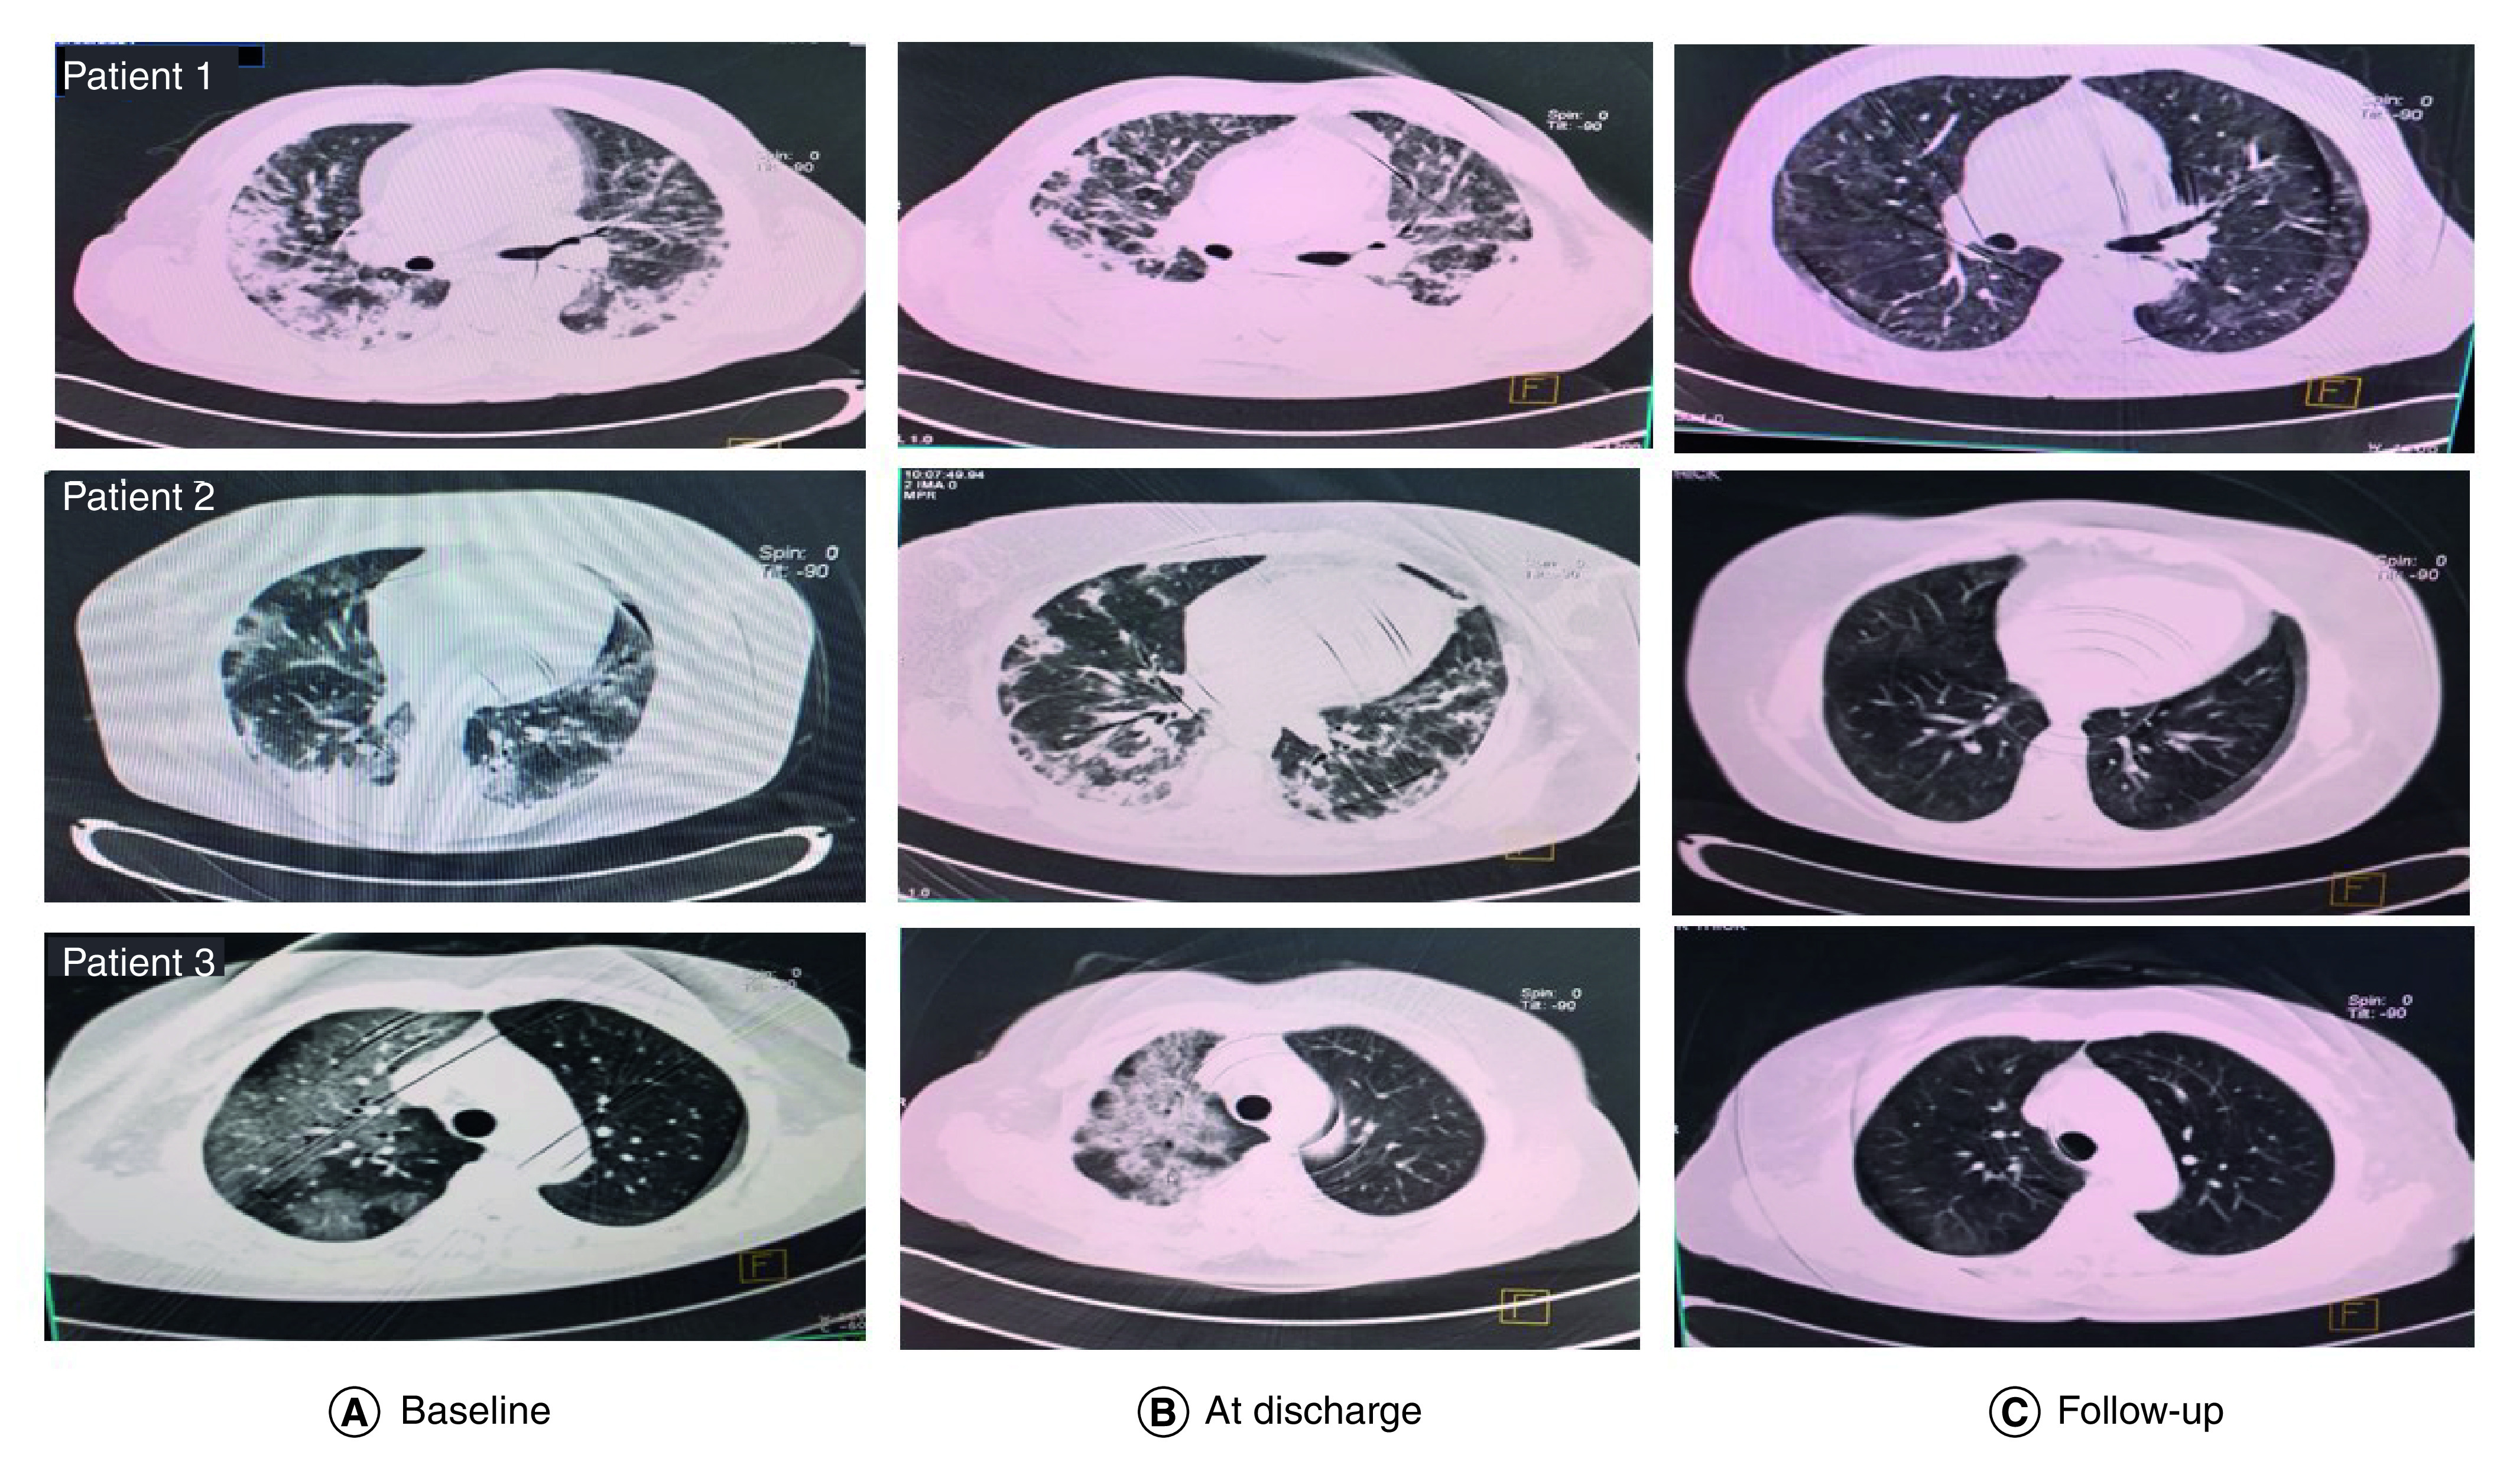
Axial chest CT scans of three patients treated with nimotuzumab. Sequential images on admission (column A), at discharge (column B) and follow-up (30–60 days after discharge). (A & B) Extensive areas of ground-glass opacities, airspace consolidation in exudative phase and decreased lung volumes in organizing and fibrotic phases. (C) Follow-up: the three patients showed resolution of the lung inflammatory lesions and no sign of fibrosis.
